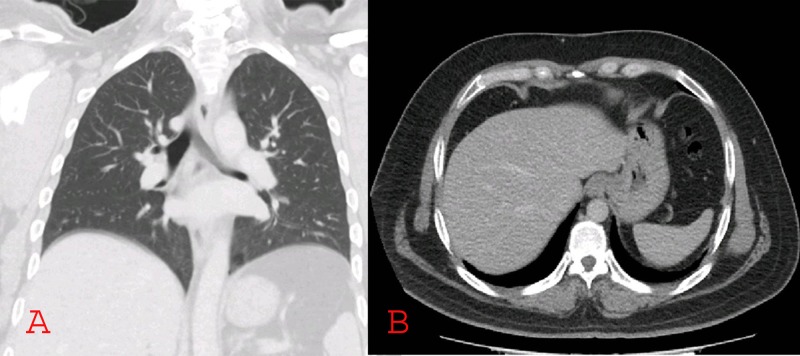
Computed tomography scan showing normal lungs (A) and liver (B)
